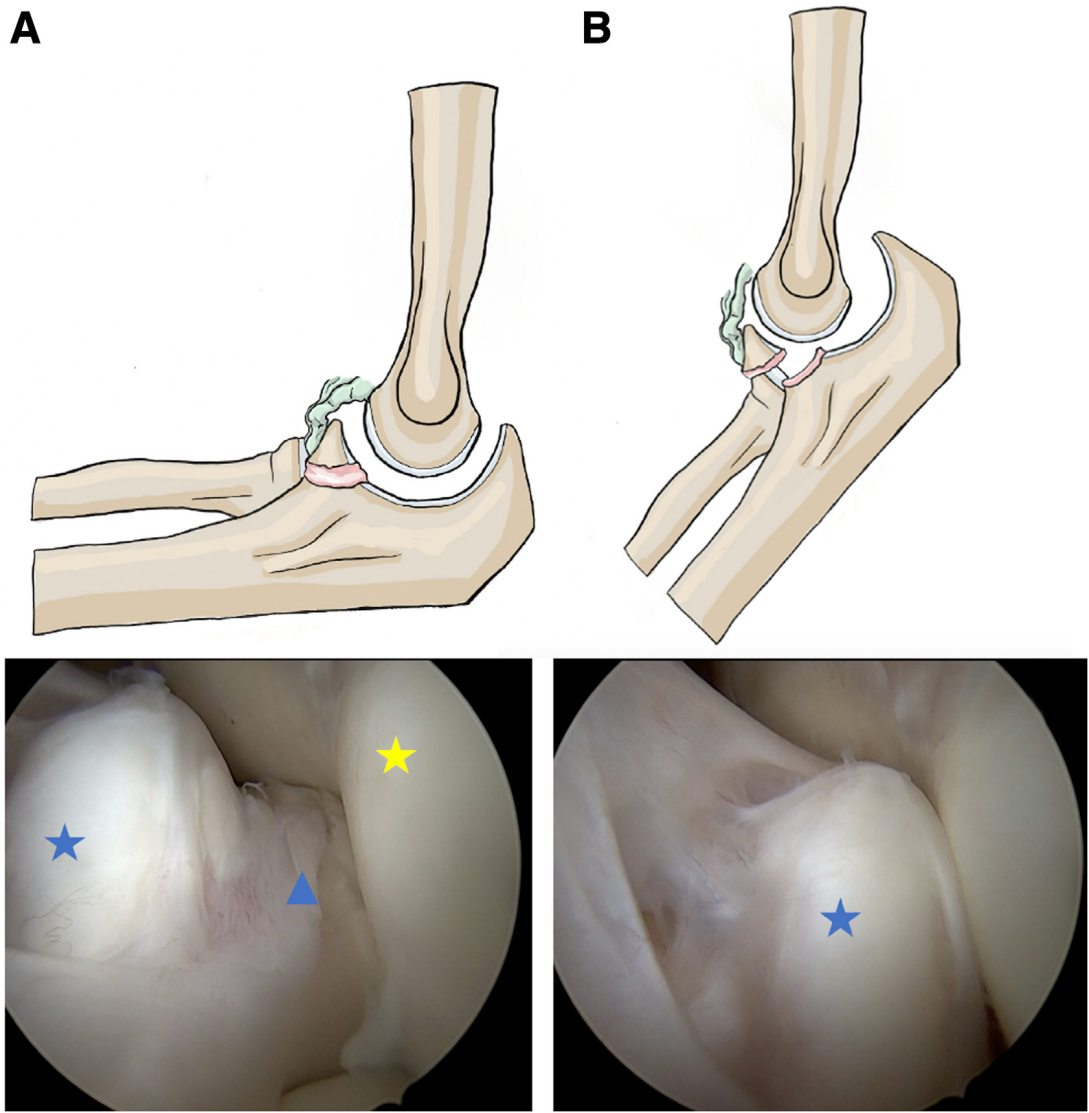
Radiographic assessment of a coronoid process nonunion and posterior displacement of the ulna in a left elbow (lateral view). (A) Persistent coronoid process nonunion is shown. (B) Posterior ulnar subluxation is shown. The patient is positioned supine with the elbow in lateral projection. Blue star: coronoid process; blue triangle: ulnar base; yellow star: humeral trochlea. This assessment confirms joint instability and the need for surgical intervention.
